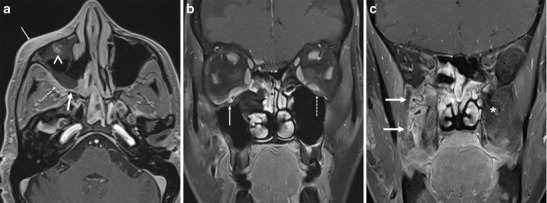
a Perineural spread of infection along the maxillary division (V2) of the right trigeminal nerve caused by E. coli infection of the right maxillary sinus in a 47-year-old female, who presented with fever and hypoesthesia along the right V2 dermatome. Axial contrast-enhanced FS T1W MR image shows right maxillary sinusitis involvement of the right PPF (*thick arrow*), PMF (*dotted arrow*), and the skin and subcutaneous tissue (*thin arrow*) overlying the right maxillary region. Thickening and abnormal enhancement of the right ION (*arrowhead*) due to perineural spread of infection is evident, thus resulting in hypoesthesia along the right V2 dermatome. b Coronal contrast-enhanced FS T1W MR image of the same patient shows asymmetrical thickening and abnormal enhancement of the right ION (*solid arrow*) secondary to perineural spread of infection from the PPF. Note the normal left ION (*dotted arrow*). c Coronal contrast-enhanced FS T1W MR image of the same patient shows abnormal enhancement with increased vascularization of the right PPF (*arrows*) due to its infective involvement by the right maxillary sinusitis. The contralateral PPF (*asterisk*) shows a normal appearance
